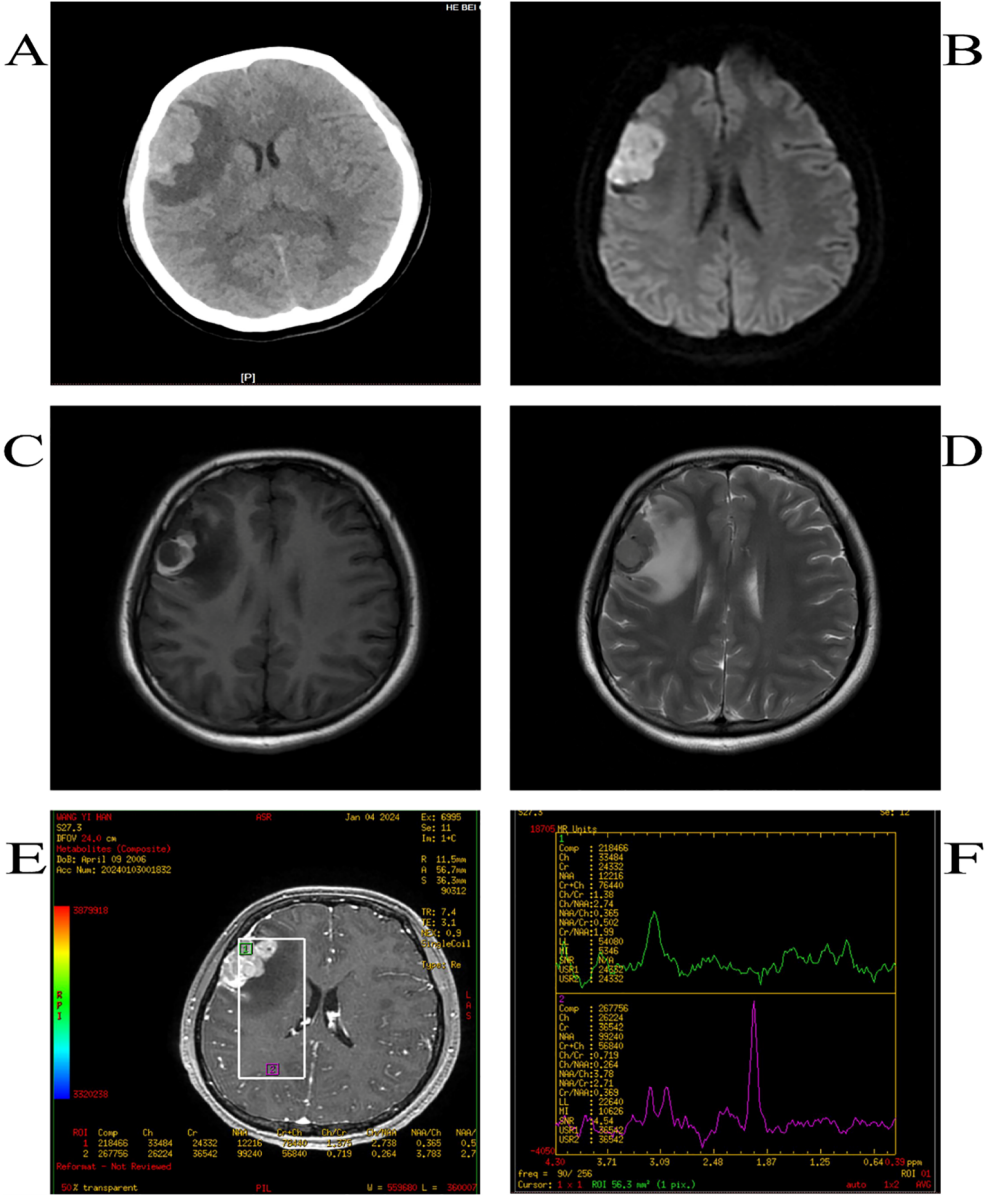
CT shows high-density opacities such as round-like images (A),DWI high signal (B),T1WI high-signal (C), T2WI low-signal shadow (D), the Cr peak was lower, the Cho peak was higher, the NAA peak was not shown, and the Cho/Cr was higher (E, F).
